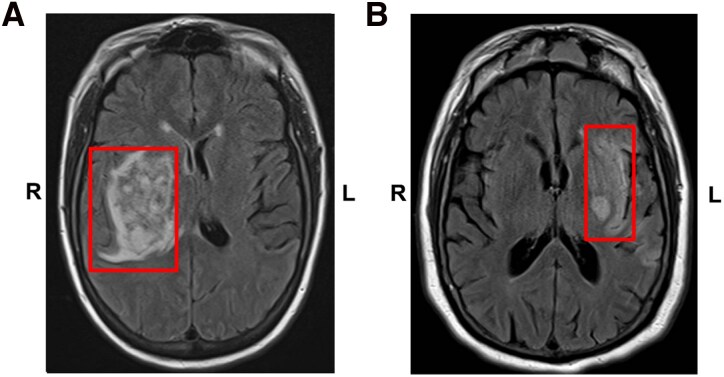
MRI scans for case examples. These T2-weighted fluid-attenuated inversion recovery (T2 FLAIR) axial slice MRI scans demonstrate the lesions from our two patient case examples, affecting the insula and basal ganglia on the right (A, Case 1) and the left (B, Case 2) hemisphere, respectively. Lesion areas are outlined in red, and ‘R’ and ‘L’ indicate the right and left hemispheres, respectively.
